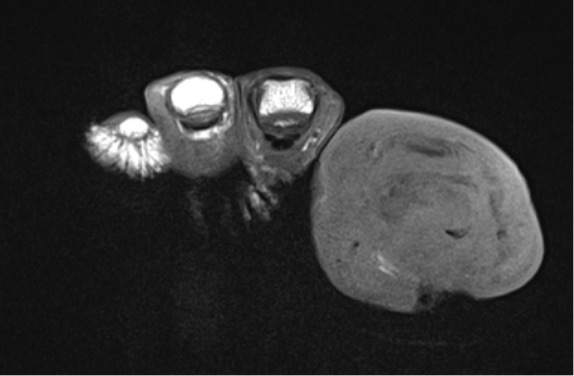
T1-weighted coronal images of the same case
